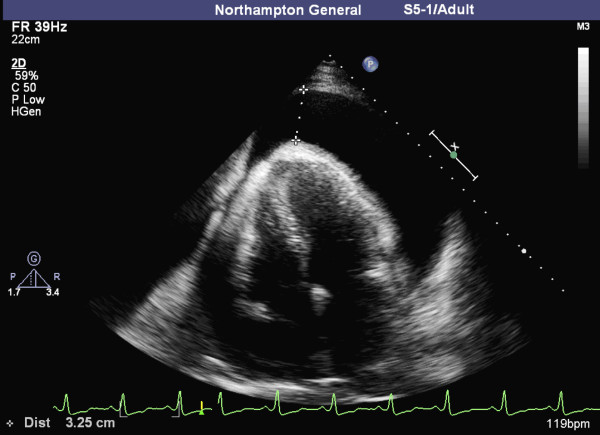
Apical four-chamber ECHO demonstrating: a 3.5 cm pericardial effusion apically (at the cursor) and more than 4 cm around the anterior wall; right atrial collapse from resulting tamponade and; although there was no evidence of left ventricular hypertrophy, the highly echogenic myocardium is also typical of Fabry's.
